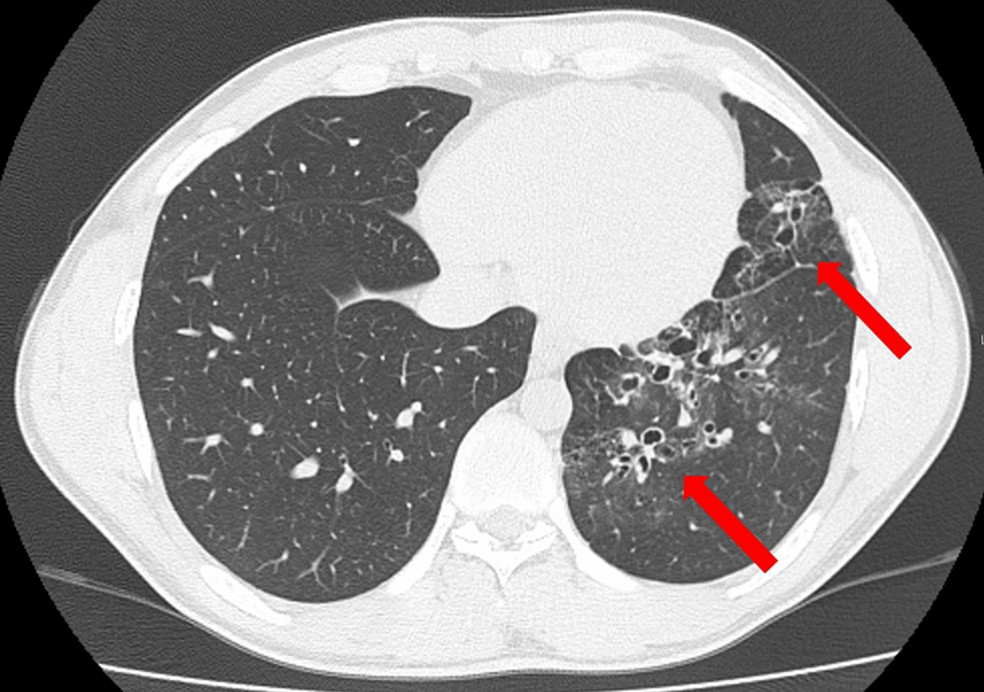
Chest CT Lung window CT revealed infiltrates, gland grass opacities and bronchodilation in the left inferior lingular segment and the left lower lobe (arrows).
